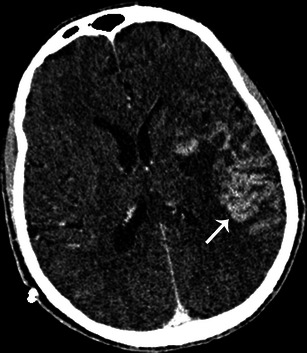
Contrast-enhanced cerebral CT following a stroke during infective endocarditis. Contrast cerebral CT shows enhancement of the cortical layer, which suggests a breakdown of the blood-brain barrier (*white arrow*)
